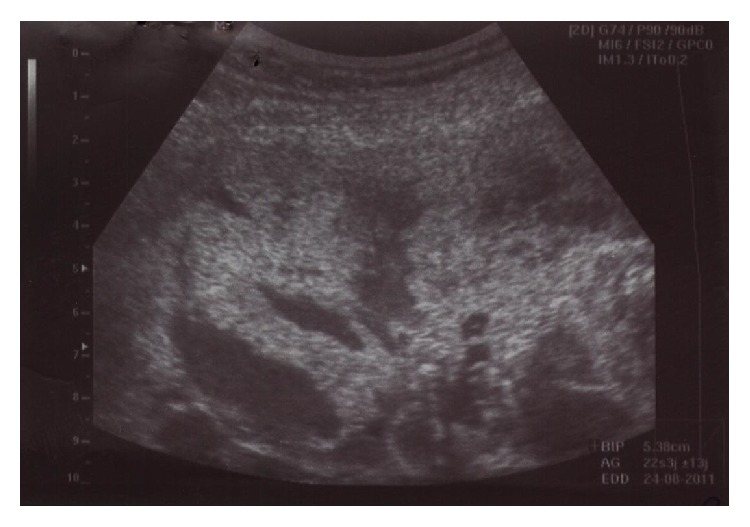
Ultrasonography appearance of a lacunary placenta.
